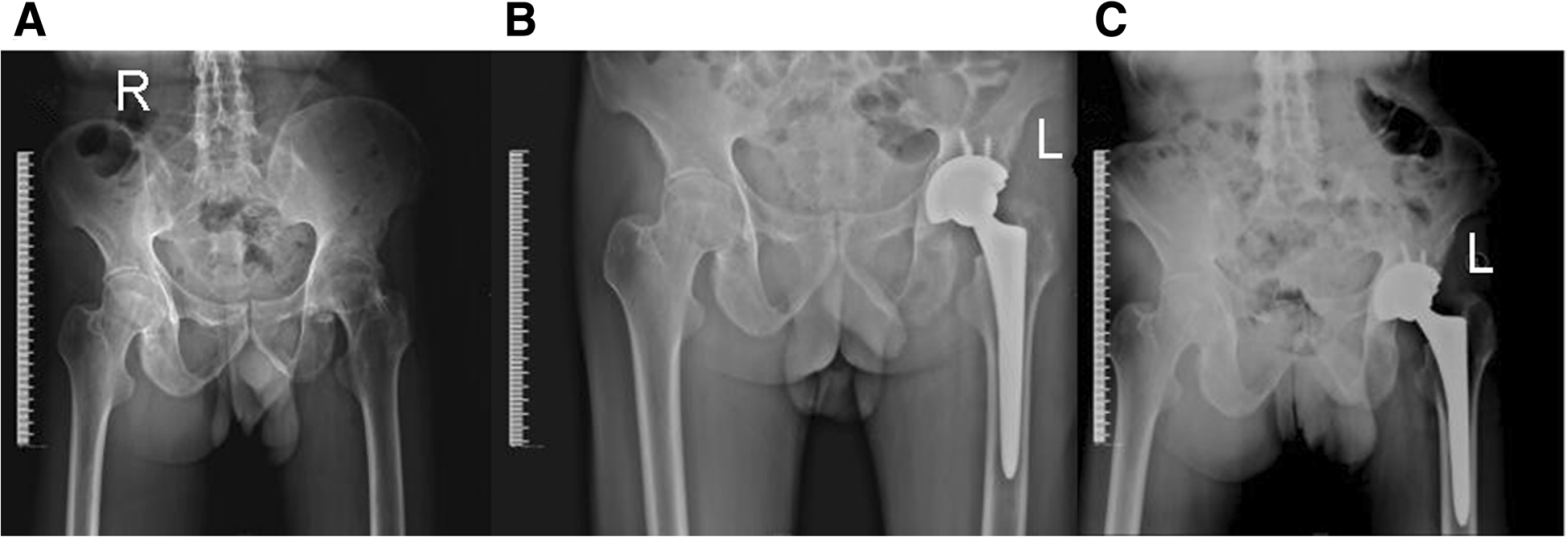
X-ray films of 1 typical cases before and after operation. a Male, 20 years old, preoperative pelvic X-ray film. b Male, 20 years old, postoperative 5 days, the pelvic X-ray film. c Male, 20 years old, postoperative 4 year pelvic X-ray film
